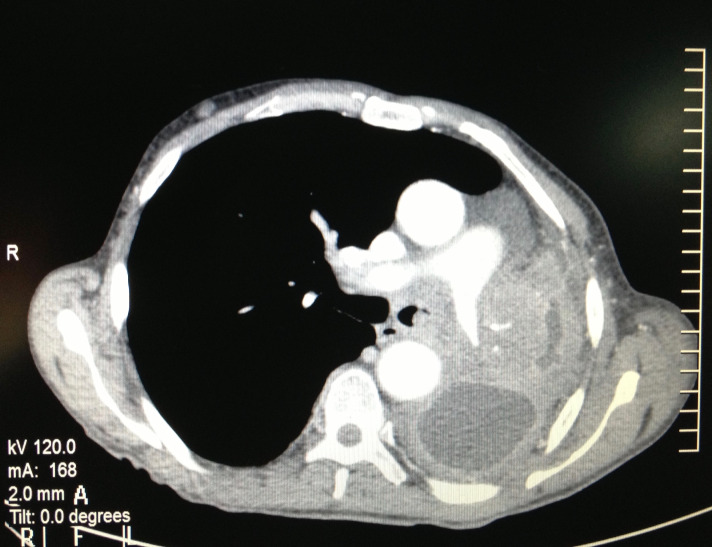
Axial computed tomography images of the chest, showing a
